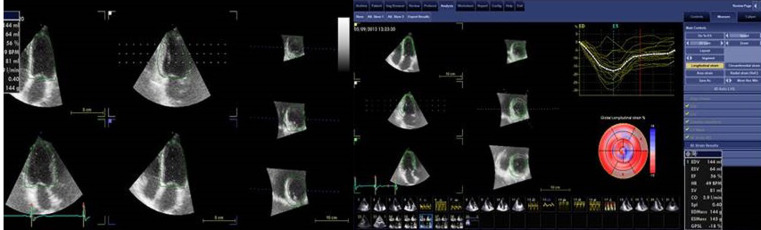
demonstration of three-dimensional speckle tracking imaging (3D-STI) analysis; after the reference points are set, the software shows the endocardial border tracking in different views; apical 4-chamber view, apical 2-chamber view, apical left ventricular longitudinal view; and three short-axis views from apex to base; the endocardial tracking is performed automatically through the entire cardiac cycle by 3D-STI
